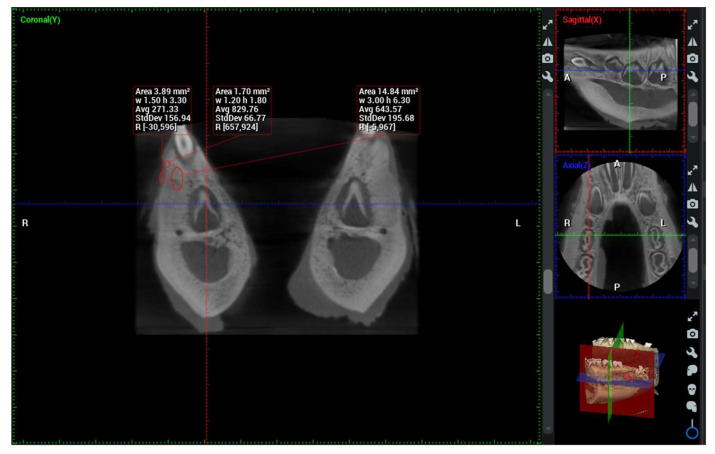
Analysis of bone density with CBCT and regions of interest (ROIs).
